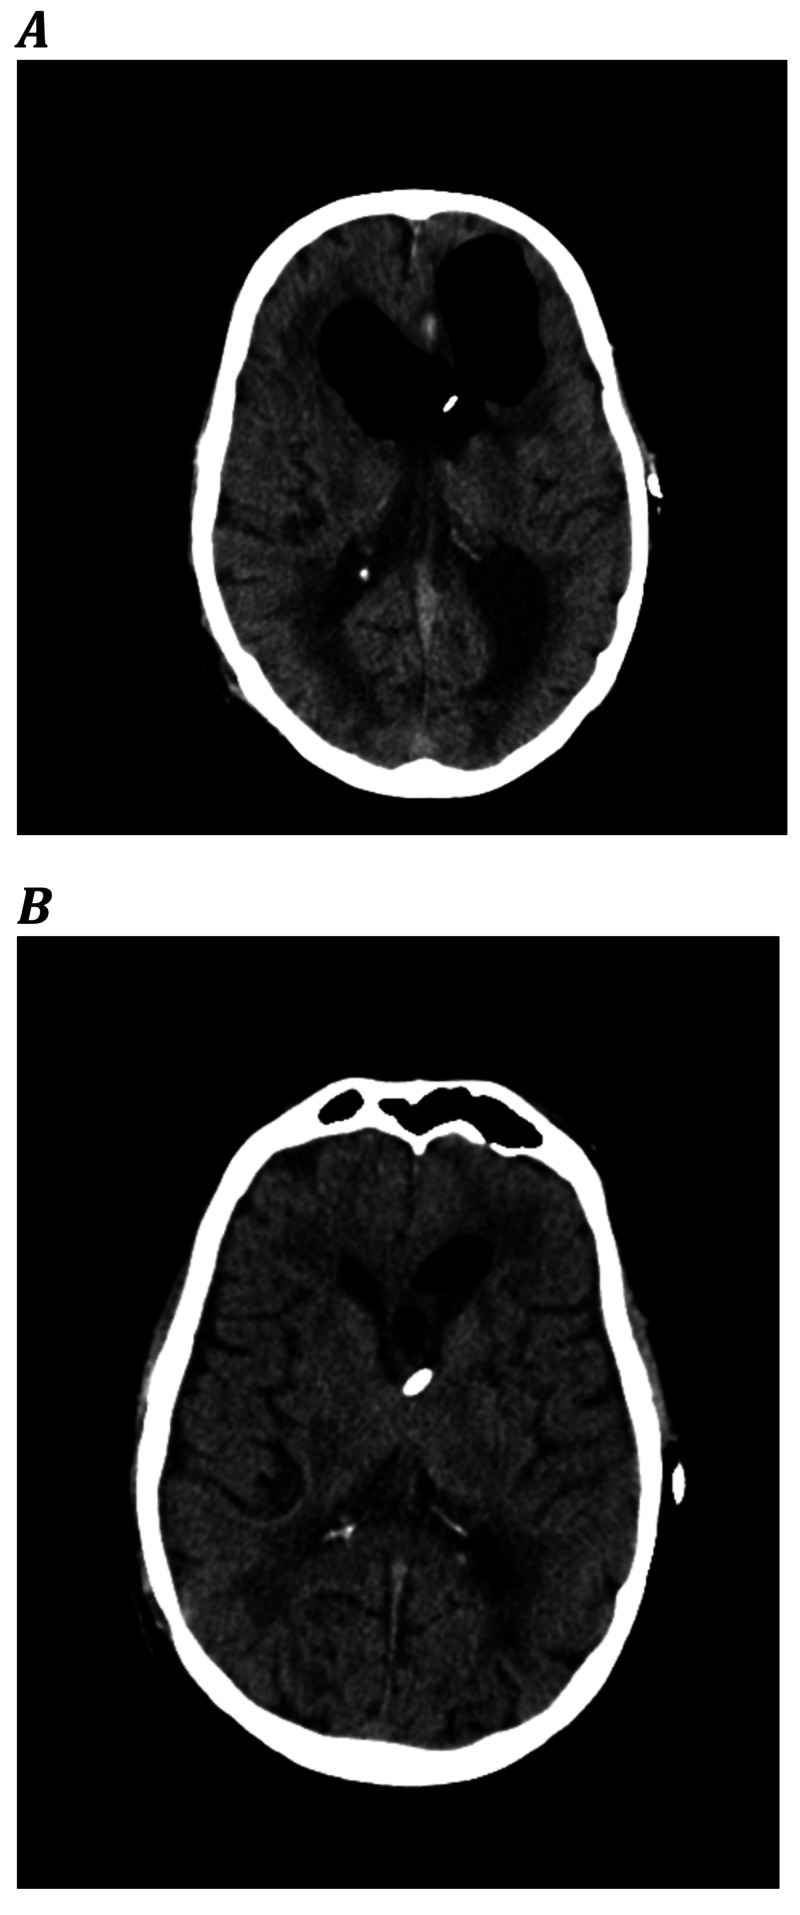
CT scans of the brain showing a pneumocephalus. A: before 100% oxygen treatment B: after 100% oxygen treatment
